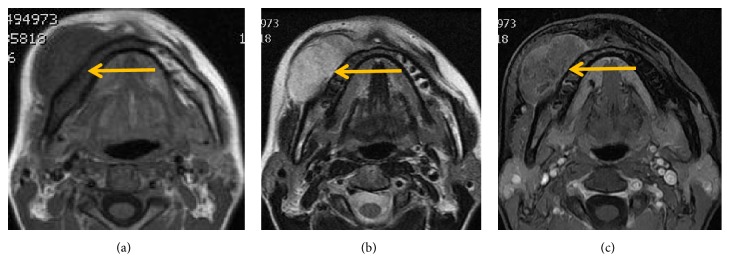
Imaging features (yellow arrow) from a 28-year-old woman of the right lower jaw. (a) T1-weighted MRI shows a uniform low signal mass. (b) T2-weighted MRI shows a heterogeneous high signal mass. (c) Gadolinium-enhanced T1-weighted fat-suppression images showed heterogeneous enhancement.
